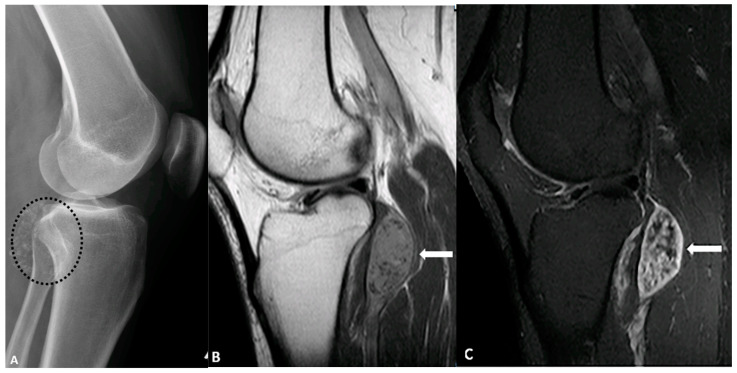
A 43-year-old female with left knee pain. (A) Lateral radiograph of the knee demonstrates multiple foci of calcification (circle) adjacent to the knee joint. (B) Sagittal T1-weighted pre- and post-contrast MRI (B,C) reveals isointense intra-articular enhancing nodules (arrows) containing non-enhancing hypointense foci, corresponding to the areas of calcification. These findings are consistent with synovial chondromatosis. The image was obtained from the University of Washington Medical Imaging database with the required permissions.
